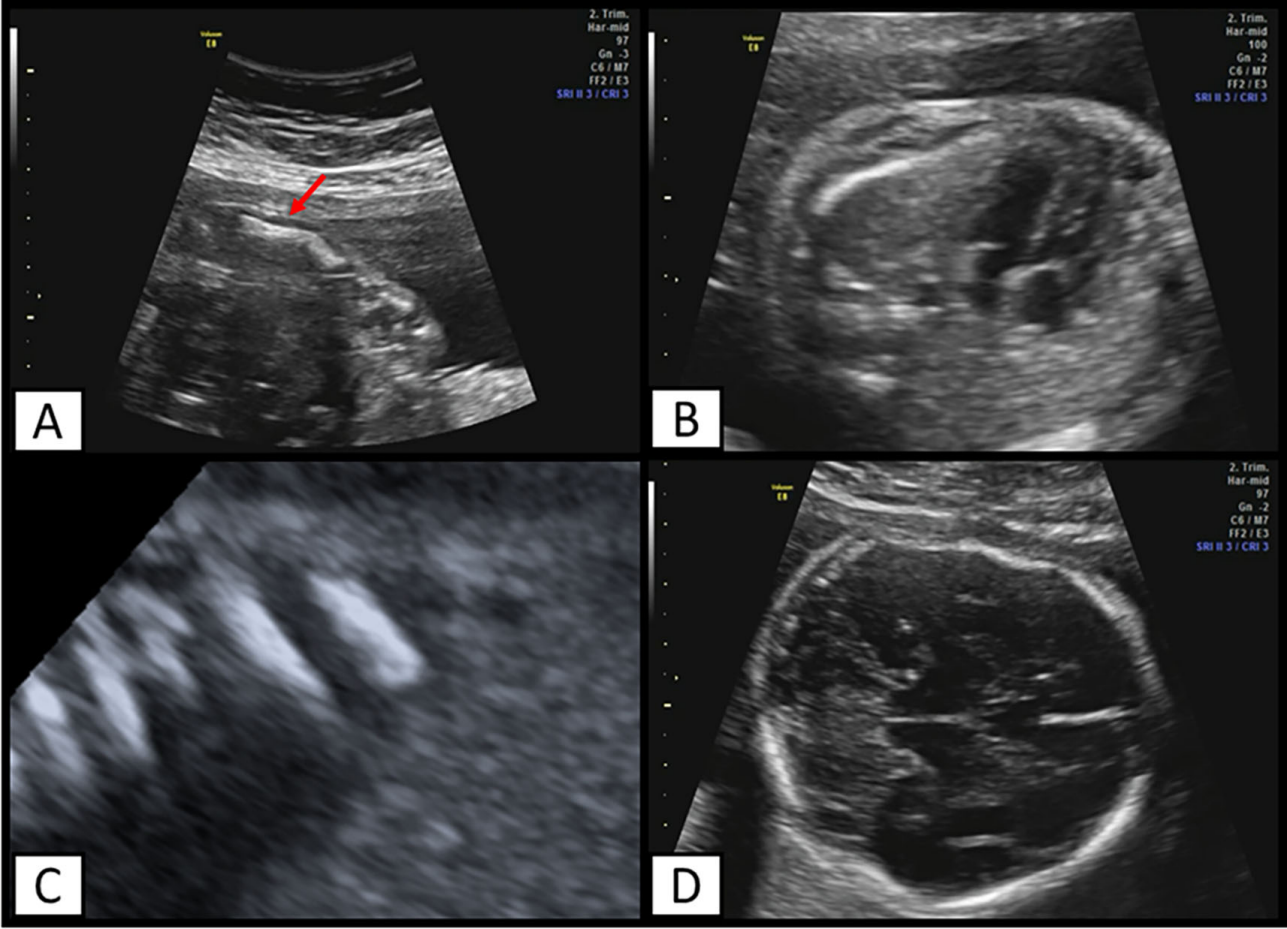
Ultrasound findings. A) Ulna with altered morphology. Arrow indicates a point fracture, B) Ribs that exceed the middle of the thorax, C) multiple rib fractures. D) Deformation of fetal head and cranial fractures can also be observed.
